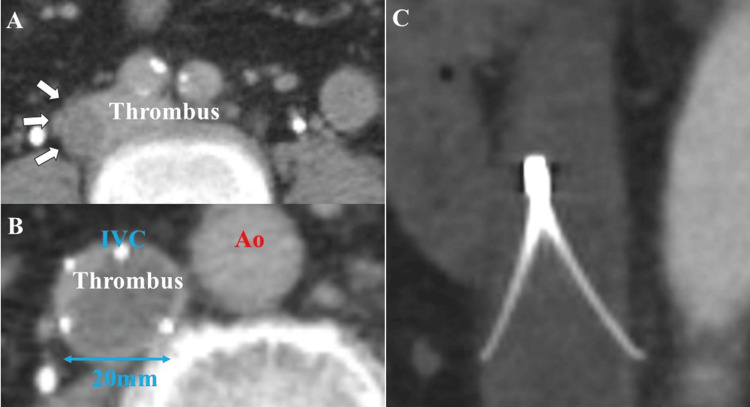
CT findings 10 days after IVC filter placement (A-B) CT findings, 10 days after IVC placement, show right iliac vein thrombosis (white arrows) extending proximally, and the IVC is occluded with a venous thrombus. The diameter of the IVC (blue double-headed arrow) is 20 mm. (C) The IVC filter is located in the IVC without any perforation. CT: computed tomography; IVC: inferior vena cava; Ao: aorta
